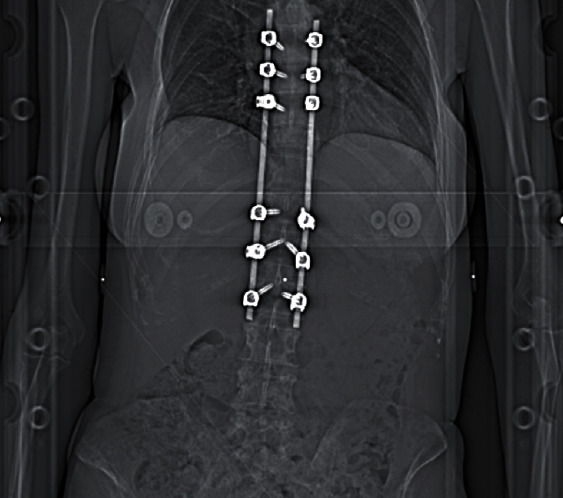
Spinal stabilisation and instrumentation construct (AP radiograph, at the time of radiotherapy).
